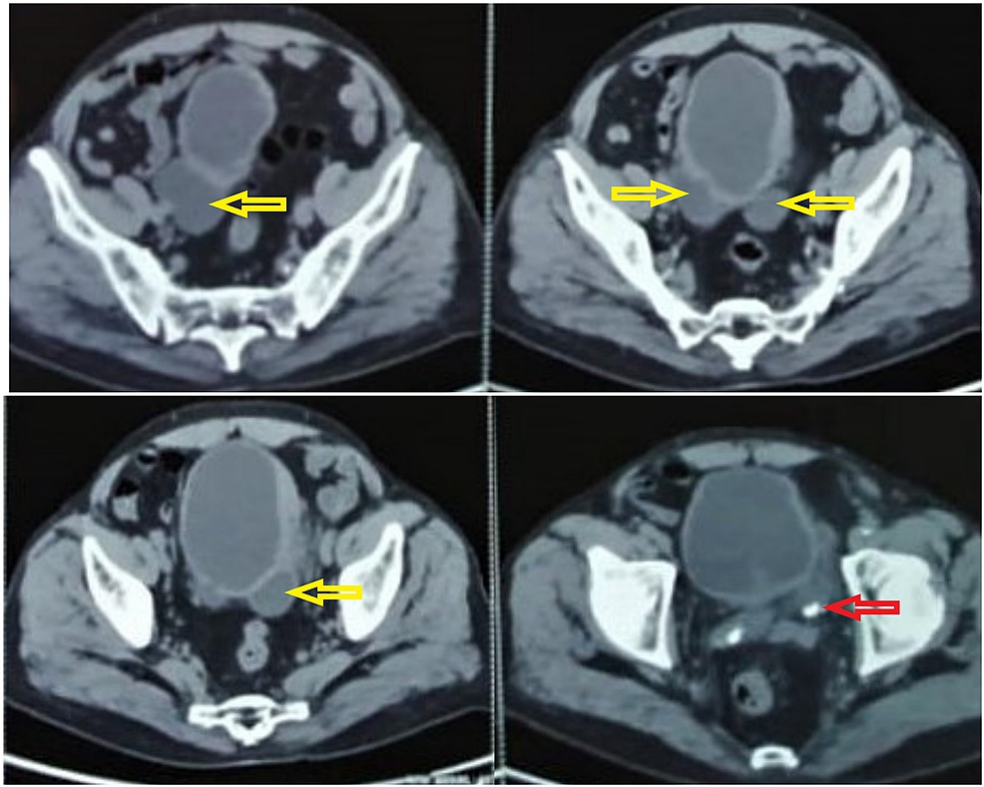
Contrast-enhanced computed tomography (CECT) scan of pelvis shows multiple urinary bladder diverticula (yellow arrows) and stone inside the diverticulum (red arrow)
